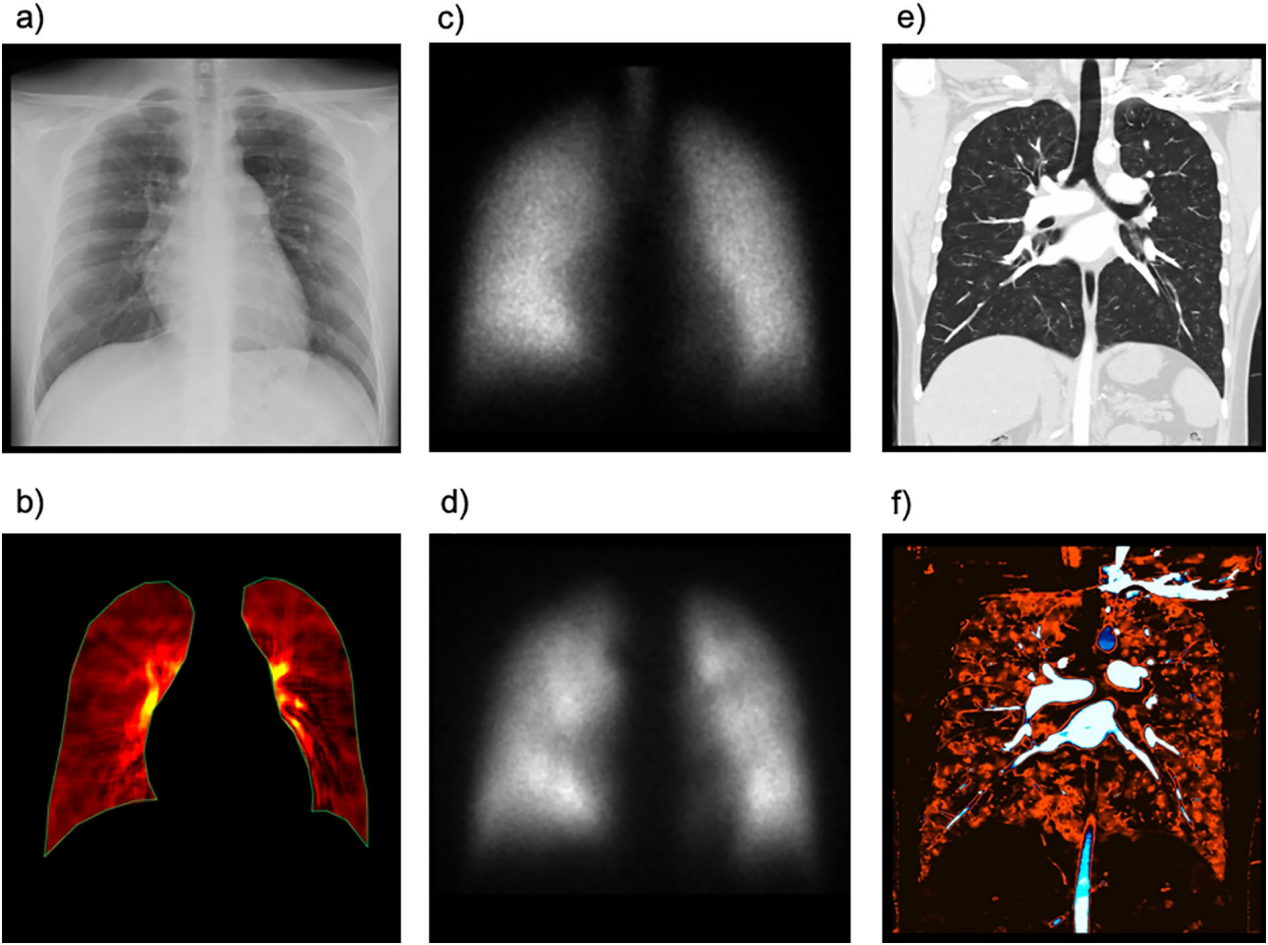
Images of a 21-year-old man with familial pulmonary arterial hypertension. Normal lung findings are shown on the chest radiograph (a) and perfusion image of the dynamic chest radiograph (b). No definite ventilation/perfusion mismatch is observed on anterior planar ventilation/perfusion scintigraphy (c, d). Chest computed tomography (CT) showing multiple subtle centrilobular ground-glass opacities in both lungs (e). Iodine images derived from CT pulmonary angiography showing slightly heterogeneous enhancement corresponding to lung opacities; however, no embolic-type defect was observed in either lung (f)
